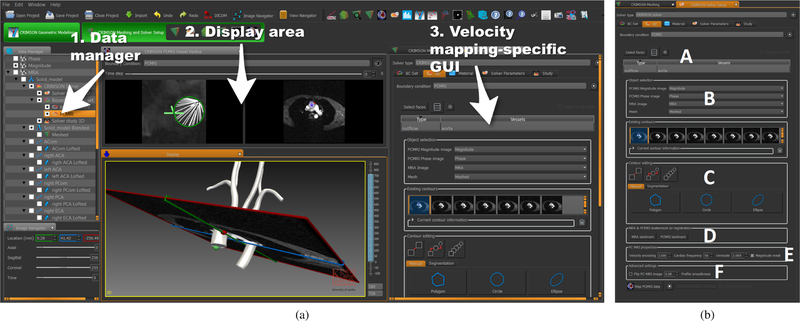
CRIMSON GUI overview. a) 1. Data manager with all the loaded objects, 2. Display area used for data visualisation, and 3. Activity-specific GUI controls are located on the right side. In this example the selected activity is velocity profile mapping. b) Detailed view of the CRIMSON velocity profile mapping GUI, which includes: A) Model face selection. B) Specification of velocity and anatomy images and geometric model. C) Vessel lumen segmentation in the velocity images. D) Positioning of landmarks in both model and velocity images. E) Additional information related to the velocity image in the context of PC-MRI (such as velocity encoding). F) Advanced settings.
